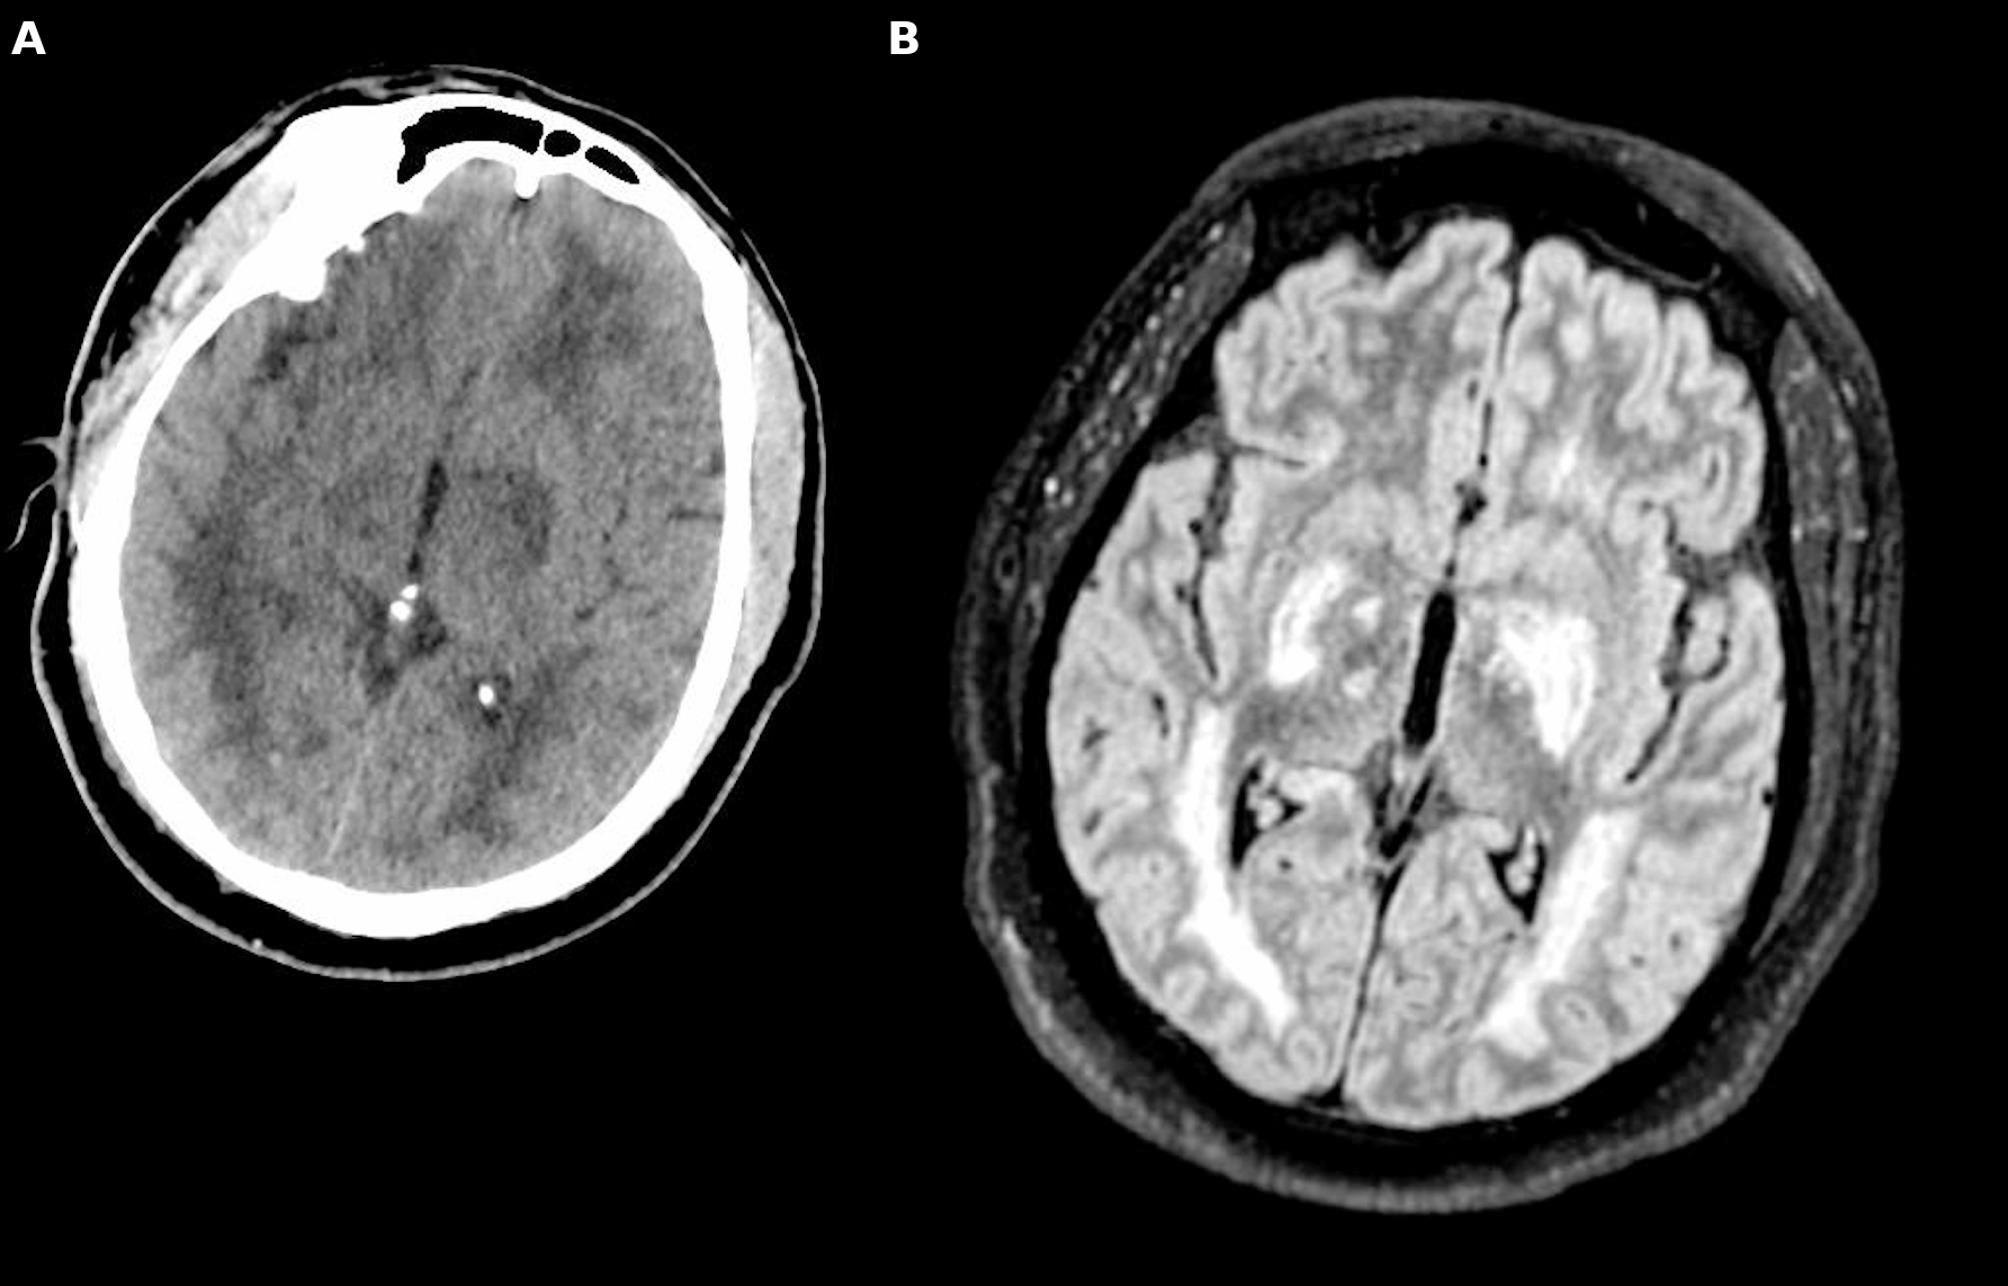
Brain imaging in cerebral malaria. Cerebral CT-scan (A) and Magnetic Resonance Imaging (MRI) Fluid Attenuated Inversion Recovery (FLAIR - B) sequences showing diffuse involvement of the white matter, thalamus and globus pallidus, in a patient with cerebral malaria
